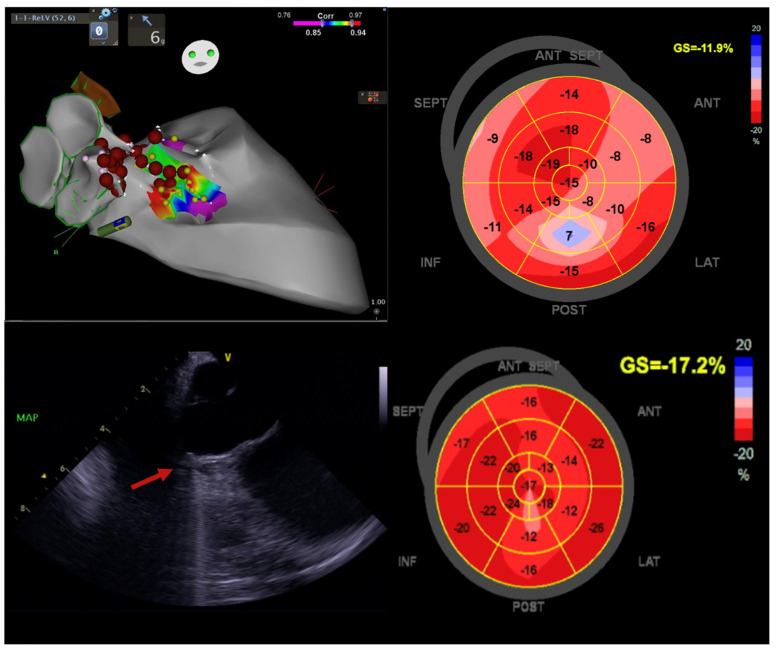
Image emphasising the significance of PVC ablation in a 60-year-old male diagnosed with HFmrEF. Initially, his left ventricular ejection fraction (LVEF) percentage stood at 42%, and his global longitudinal strain (GLS) measured −11.9%. The baseline PVC burden was noted at 22%, with PVCs localised to the right para-Hisian region. Intracardiac echocardiography unveiled a basal septal scar (indicated by a red arrow) positioned just below the right coronary cusp (RCC). At the one-year follow-up, the PVC burden plummeted to 1.2%, accompanied by an improvement in LVEF to 66% and GLS to 17.2%. This case highlights the pivotal role of PVC ablation in ameliorating left ventricular function and enhancing quality of life. Image courtesy of the Canberra Heart Rhythm Centre.
